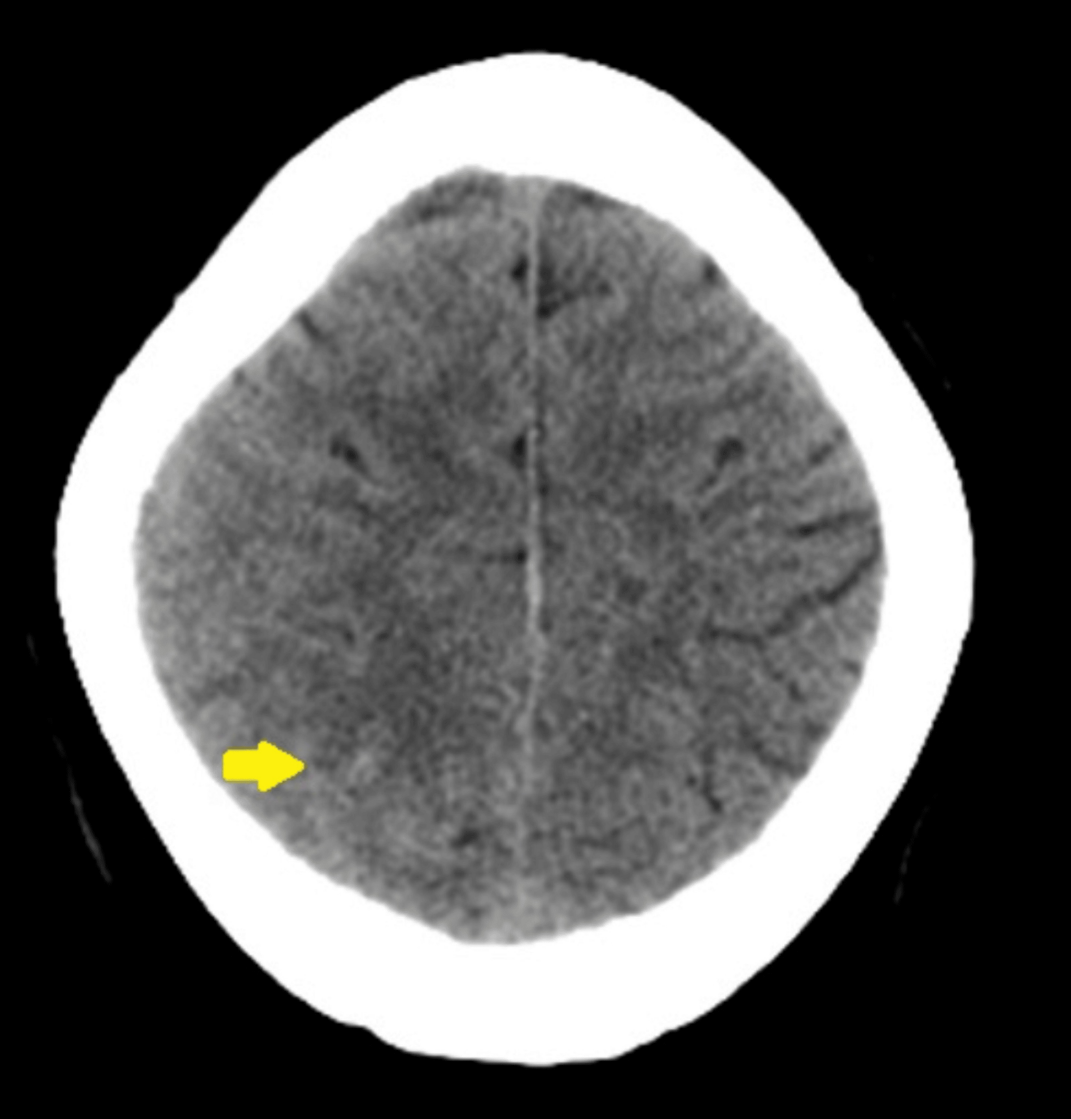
Axial non-contrast enhanced cranial CT scan taken one-day post-ictus. The CT scan revealed acute convexal subarachnoid hemorrhage over the right parietal lobe (arrow).
